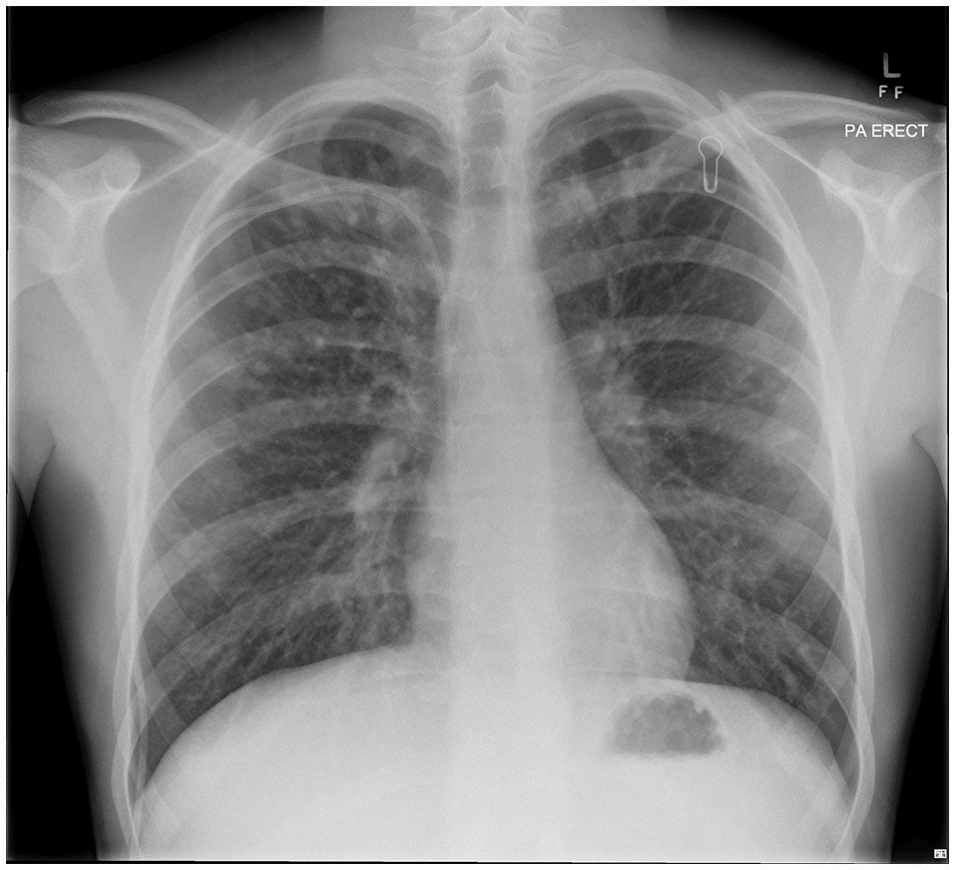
Chest x-ray, prior to commencing treatment.
